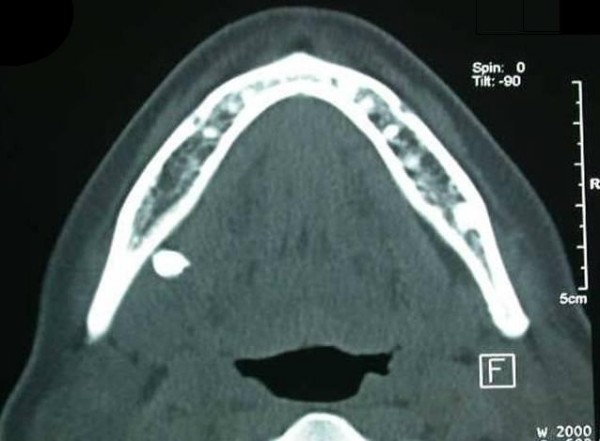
Axial Computed Tomography scan showing high density area.
